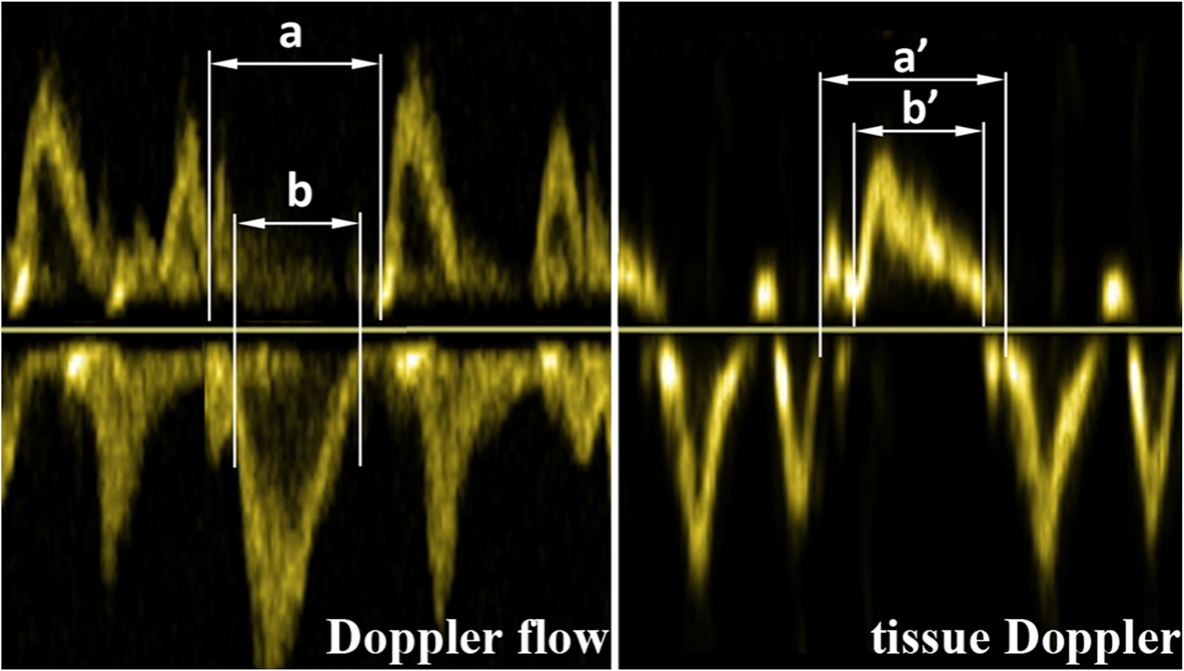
Myocardial performance index, measured by two methods: (1) Doppler flow velocity measurements of mitral inflow and left ventricular outflow tract; the index was derived by the formula: MPI = (a-b)/b, and (2) Pulsed-wave tissue Doppler measurements of mitral annulus velocity; the index was derived from the formula: MPIt = (a’-b’)/b’.
